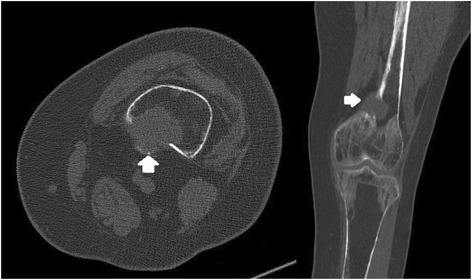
Knee CT scan: a 28*26*24 mm lobulated lytic mass in posteromedial aspect of the left distal femoral metaphysis with cortical disruption and extra osseous soft tissue formation (arrow)
